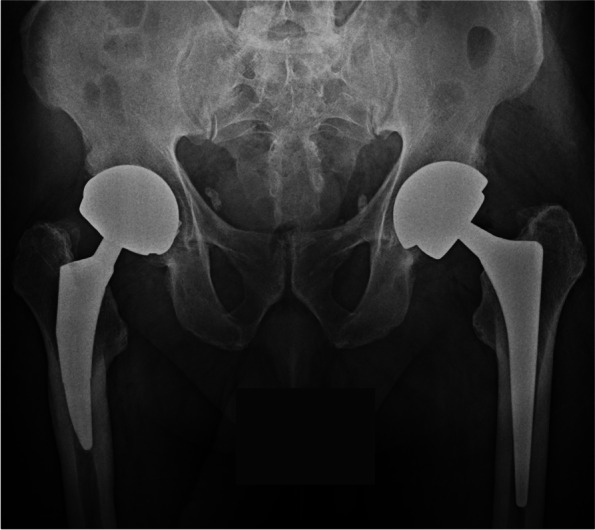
Anteroposterior pelvic radiographs after revision of the left acetabular component with a new Maxera component 4mm larger (bearing 48 mm)
